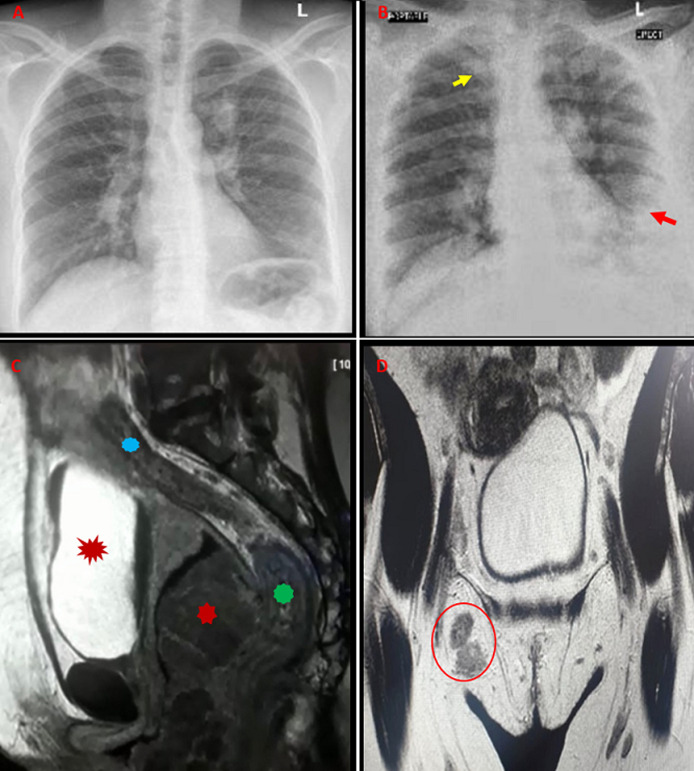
A) initial normal appearing CXR; B) metastasis evidenced by upper lobes nodular infiltrate and pleural effusion; this CXR was performed after 1 month of surgery; C) pelvic MRI reveals an ill-defined vulvovaginal tumour not involving the bladder, uterus or rectum; D) CT scan show right inguinal lymphadenopathy
